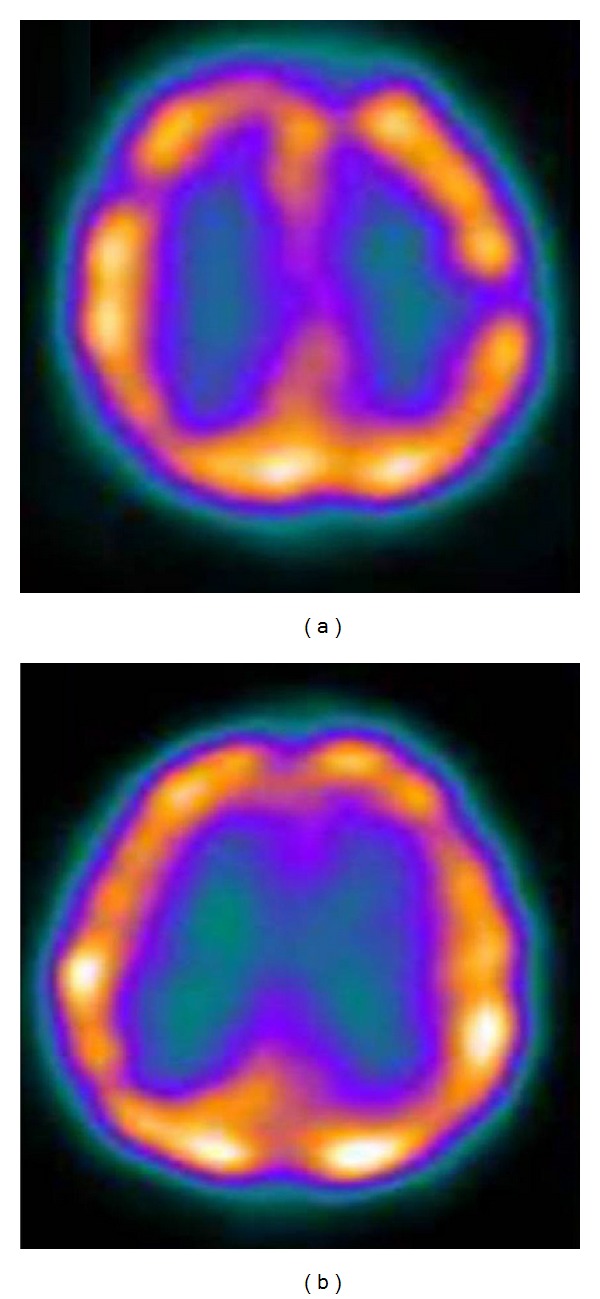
^99m^Tc-ECD brain SPECT transaxial image (a) preoperatively showing reduced radiotracer uptake involving left parietal lobe suggestive of hypoperfusion, (b) postoperatively showing increased radiotracer uptake involving left parietal lobe suggestive of improved perfusion.
